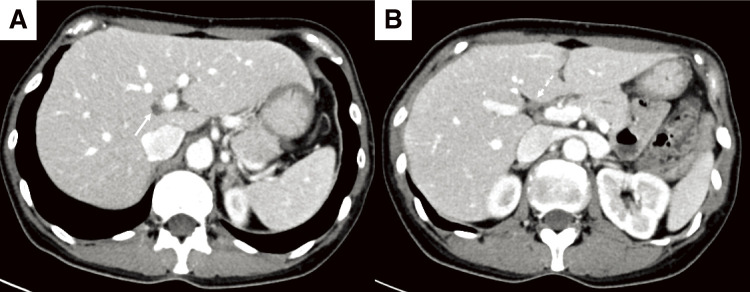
Enhanced CT findings after chemotherapy. (A, B) Both the liver tumor (arrow) and lymph nodes (dotted arrow) shrank but did not disappear. CT, computed tomography
